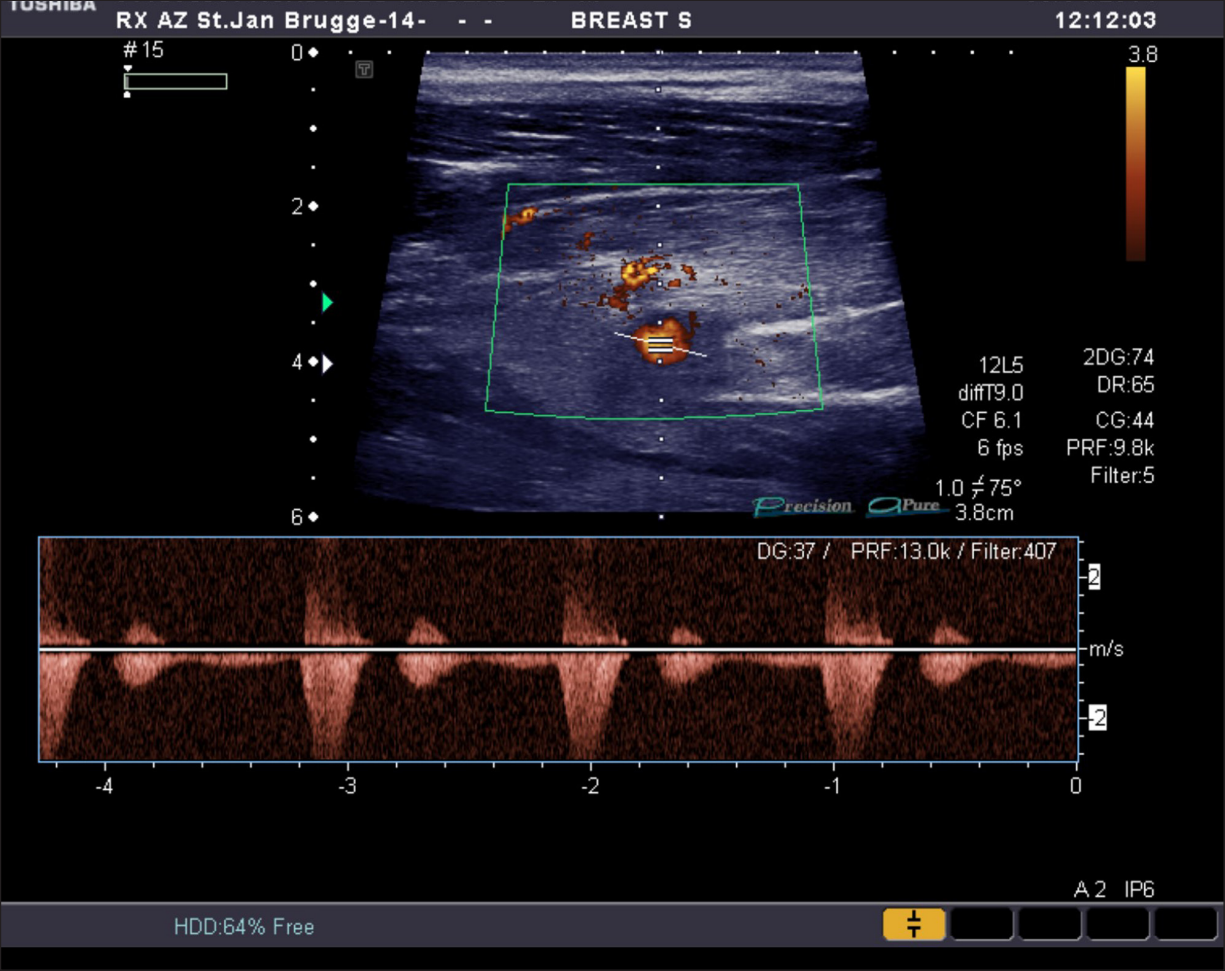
Doppler US: Arterial signal within the hematoma.
